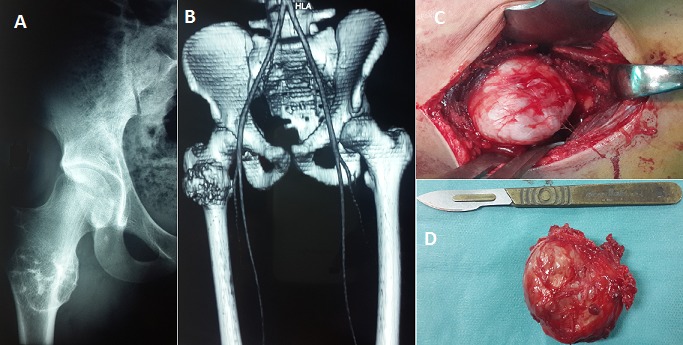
(A) radiographie standard montrant la tumeur; (B) image TDM montrant la localisation inter-trochantérienne; (C) vue per-opératoire montrant la tumeur; (D) photo de la pièce opératoire
